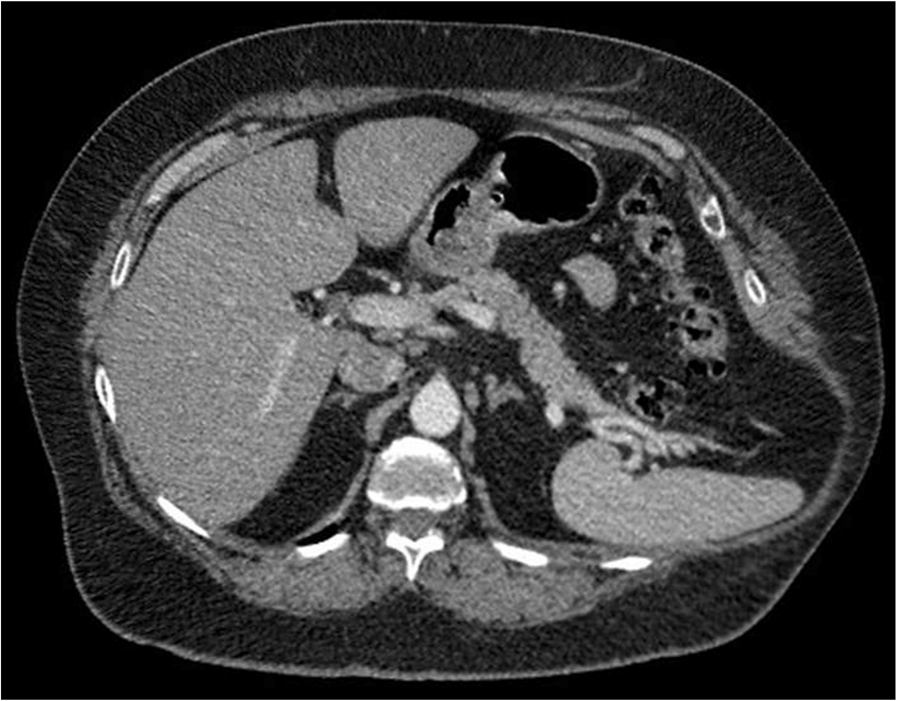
Axial contrast-enhanced computed tomography image. The image shows a bulge in the left posterolateral abdominal wall with fascial layers intact
